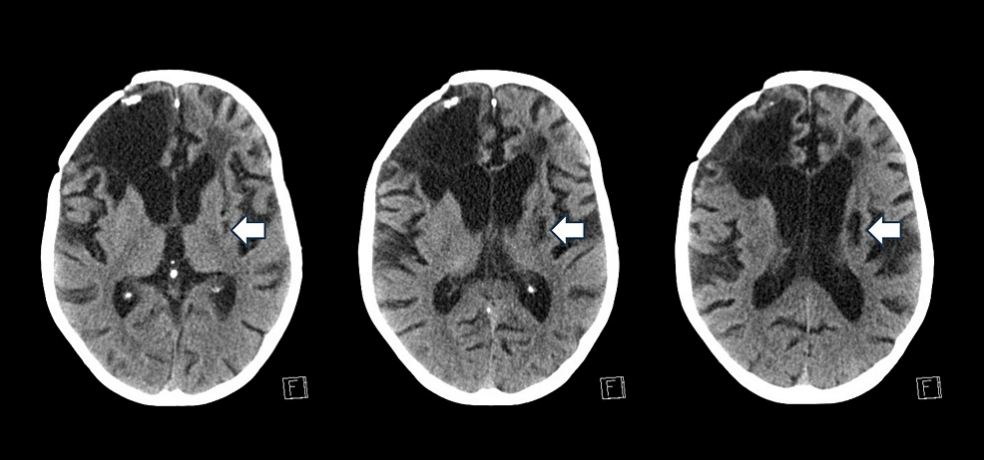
CT brain showing old infarction in the left corona radiata and basal ganglia hypodensities extending to the left external capsule with associated ipsilateral Wallerian degeneration (arrows)
